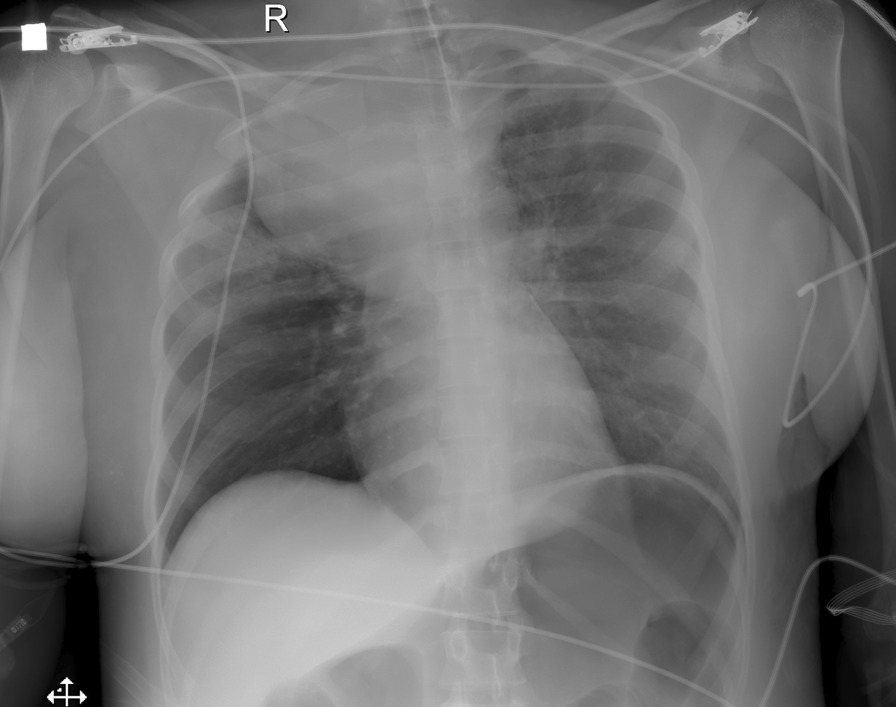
Chest X-ray showing a homogeneous paratracheal superior right mass, displacing the trachea to the left, with a well-defined inferior border. Infiltrates in the right superior lobe, adjacent to the mass. The orotracheal tube was correctly positioned. Other monitoring elements and distension of the gastric chamber are identified
